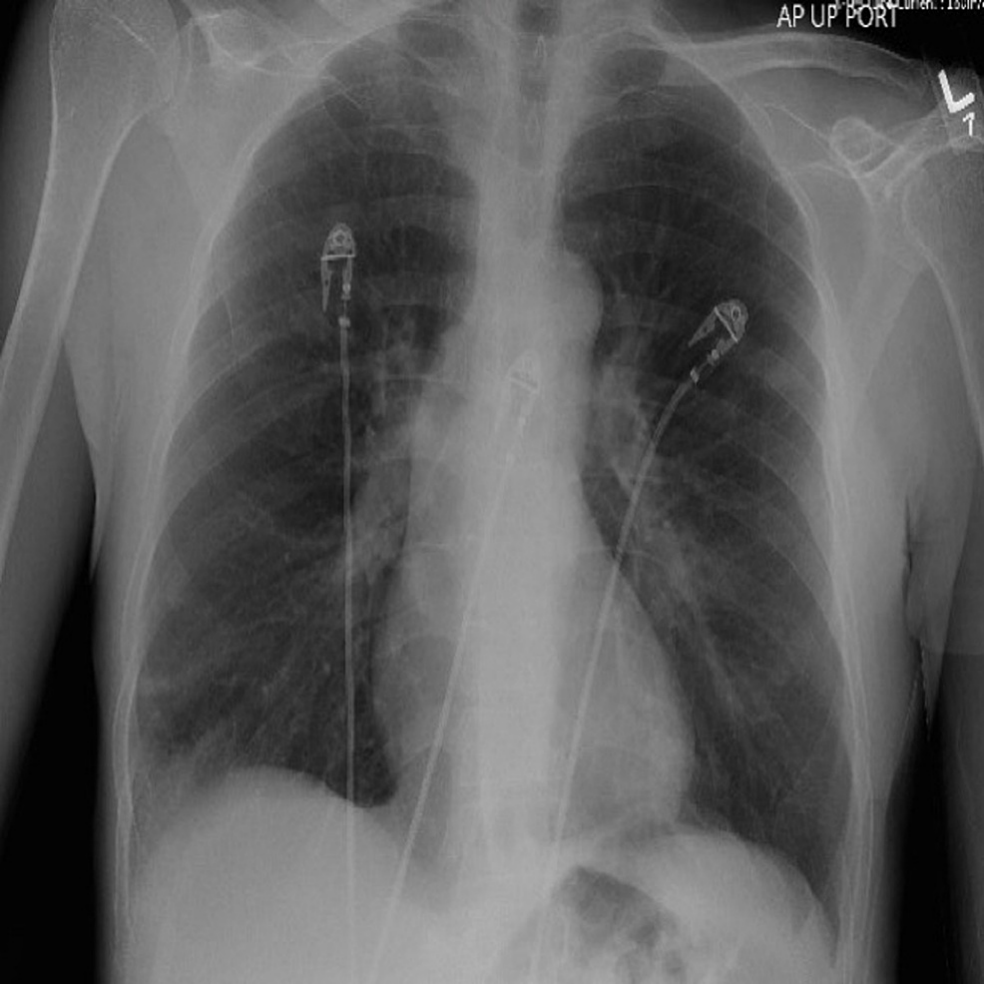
Bilateral nodular opacities on chest radiography
